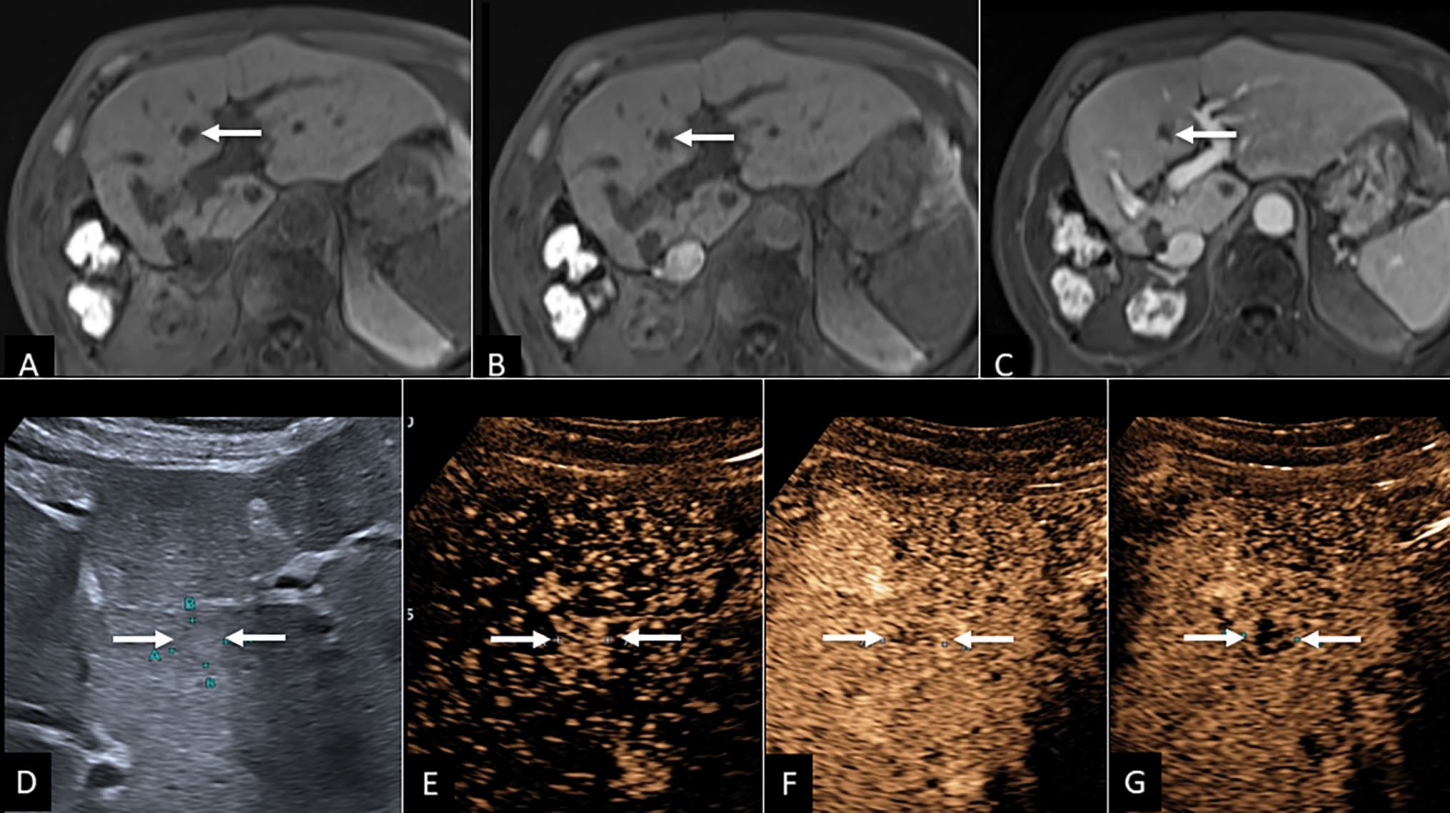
HCC with MRI LR-NC categorization. A Pre-contrast MR image demonstrating small hypo-intense observation (arrow). Arterial phase image (B) demonstrated no enhancement, likely due to mistiming of arterial phase, with persistent hypointensity on late phase image (C). D Grayscale ultrasound image showing small nodule measuring 1.1 cm with clear APHE (E). CEUS representative image at 1 min (F) showing no early washout. G CEUS representative image at 3 min demonstrating late and mild washout—typical appearance of HCC (LR-5), confirmed on follow-up MRI
